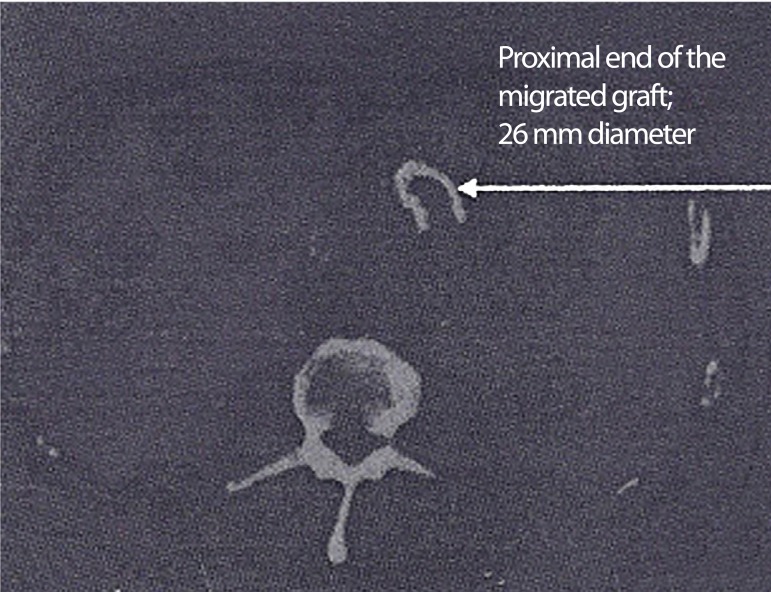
Pre-operative computed tomography (CT) scans: proximal end of migrated
